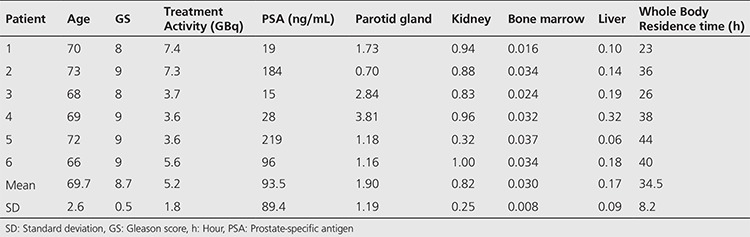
Patient characteristics and calculated radiation absorbed doses (Gy/GBq of Lu-177-PSMA-617) per organs
